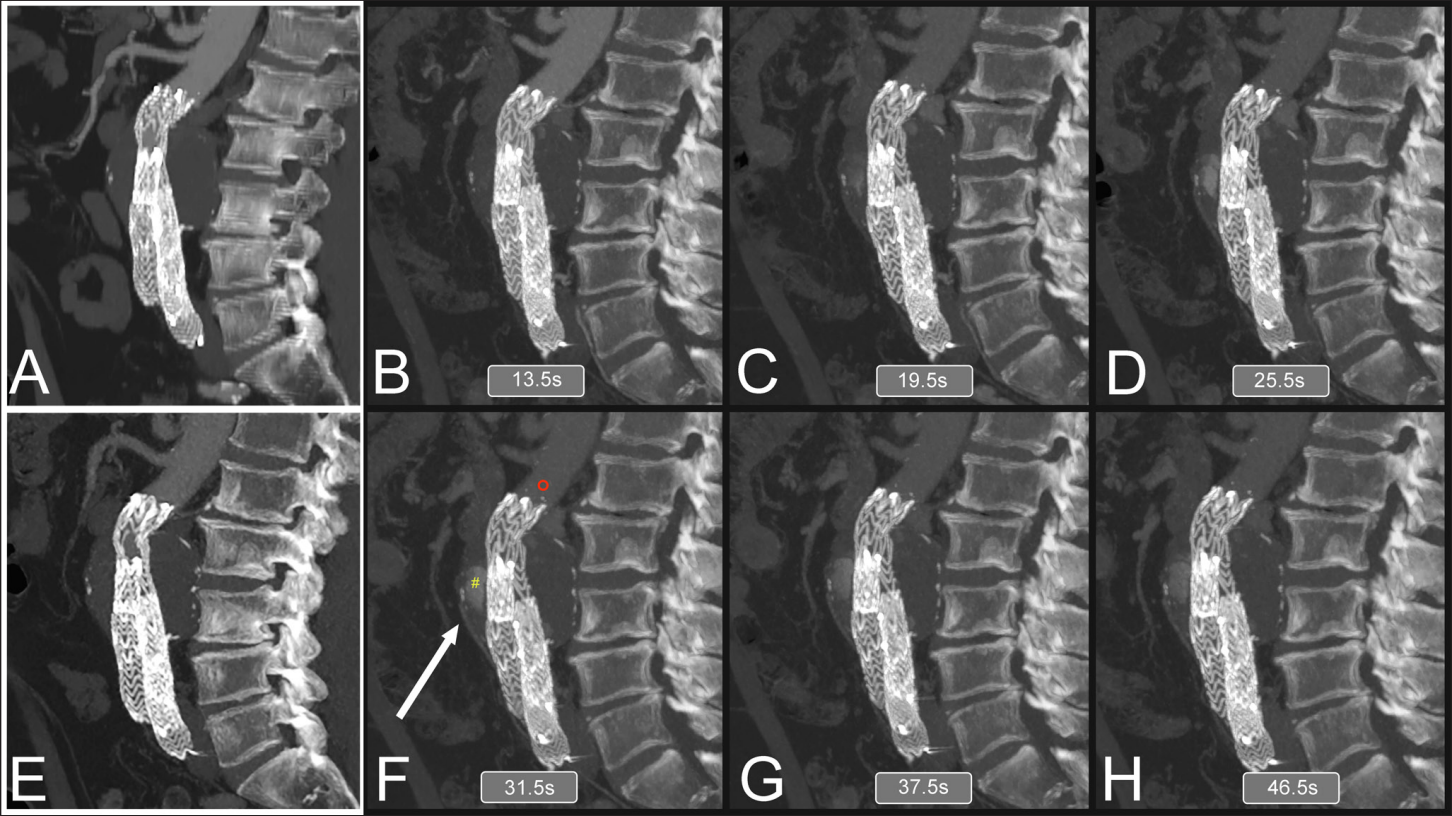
Comparison of arterial and delayed phases of triphasic computed tomography (CTA) with dynamic CTA findings. Compared to triphasic CTA, dynamic CTA is superior in identifying endoleak following endovascular aneurysm repair (EVAR). In this particular case, neither the arterial (A) nor the delayed (2 E) CTA phases were able to support endoleak as the underlying cause of progressive aneurysm sac growth following EVAR. In contrast, dynamic endoleak (panels B-D, F-H) was capable of showing the endoleak and the supplying inferior mesenteric artery (panel F, white arrow).
